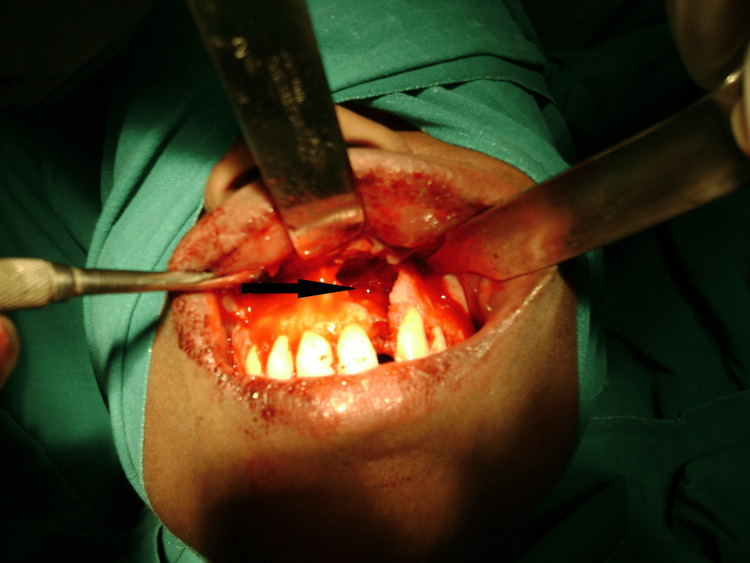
Intraoperative photograph obtained during surgical enucleation showing the cystic cavity (black arrow) following complete removal of the periapical lesion.
